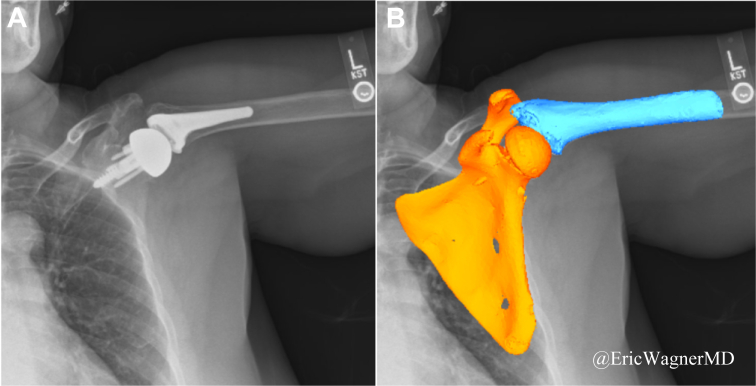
Example of 3D position and orientation of the scapula and humerus models determined using model-image registration technique in JointTrack (University of Florida, Gainesville, FL, USA) and projected onto a dynamic digital radiography (DDR) image; these 3D poses were iteratively adjusted to match their silhouettes with the silhouettes in the DDR image (A) shows the DDR image, while (B) shows the model image projected. *3D*, 3-dimensional.
